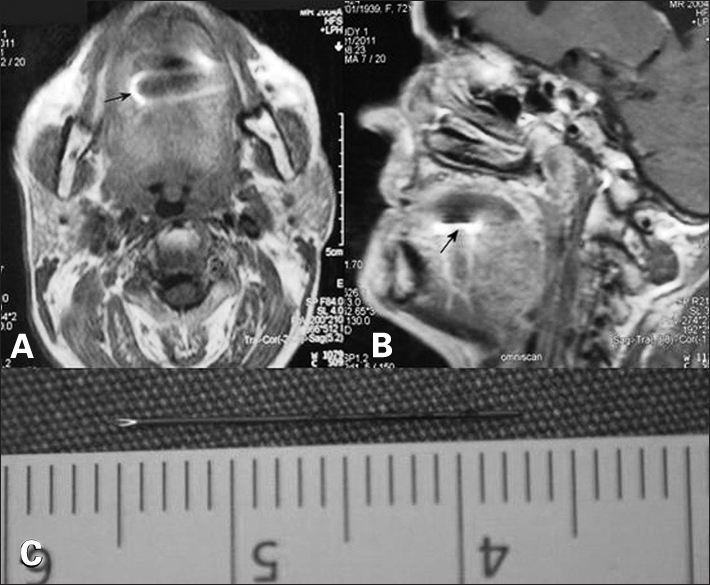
Image of the foreign body on axial (A) and sagittal (B) sections of MR imaging (black arrow). Image of the dental needle that was removed (C).
